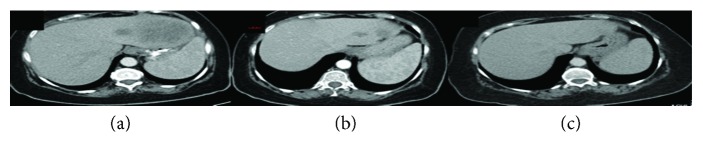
CT scan that shows liver lesions (a) before starting 1st line chemotherapy and (b) before starting immunotherapy. (c) Last CT scan showing continuous PR.
